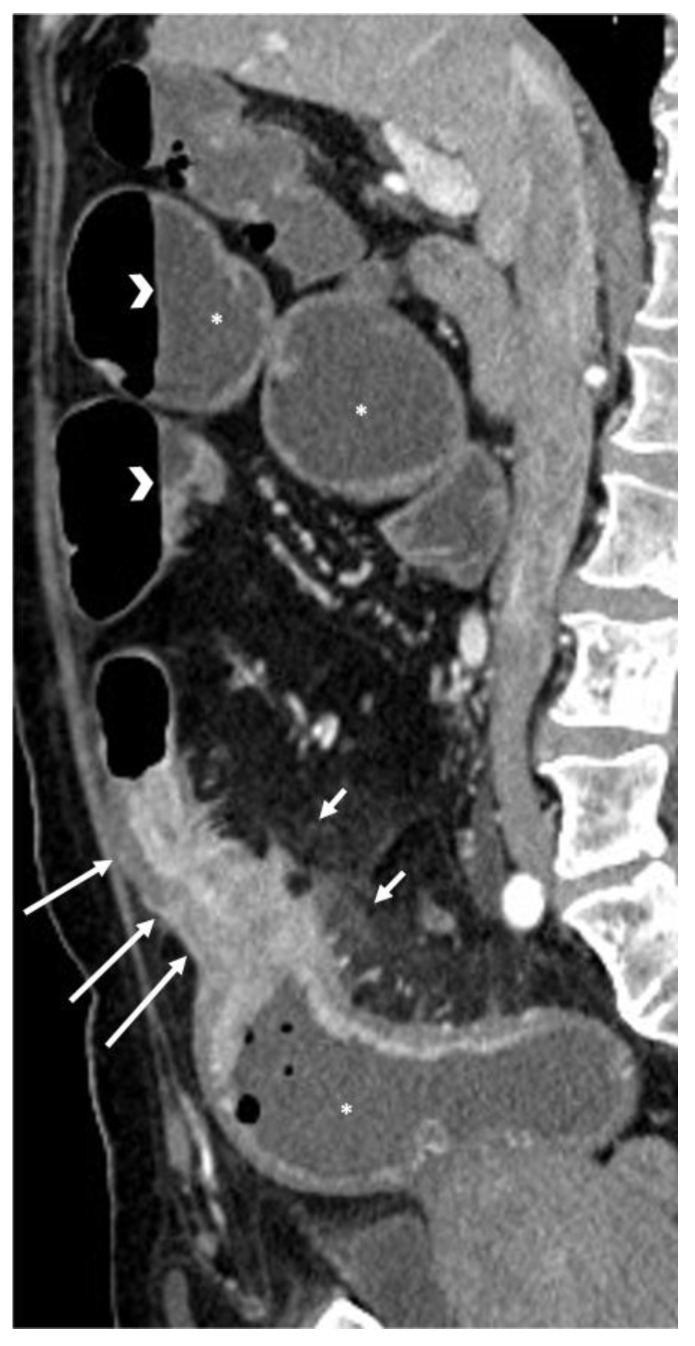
CT appearance of bowel obstruction caused by Crohn’s disease. Sagittal CT image after contrast medium injection, performed at the emergency department, shows Crohn’s disease-related bowel wall thickening (long arrows), causing upstream bowel dilatation (asterisks) with associated air–fluid levels (arrowheads). Hyperdensity of the mesentery adjacent to the bowel wall thickening is also evident (short arrows).
